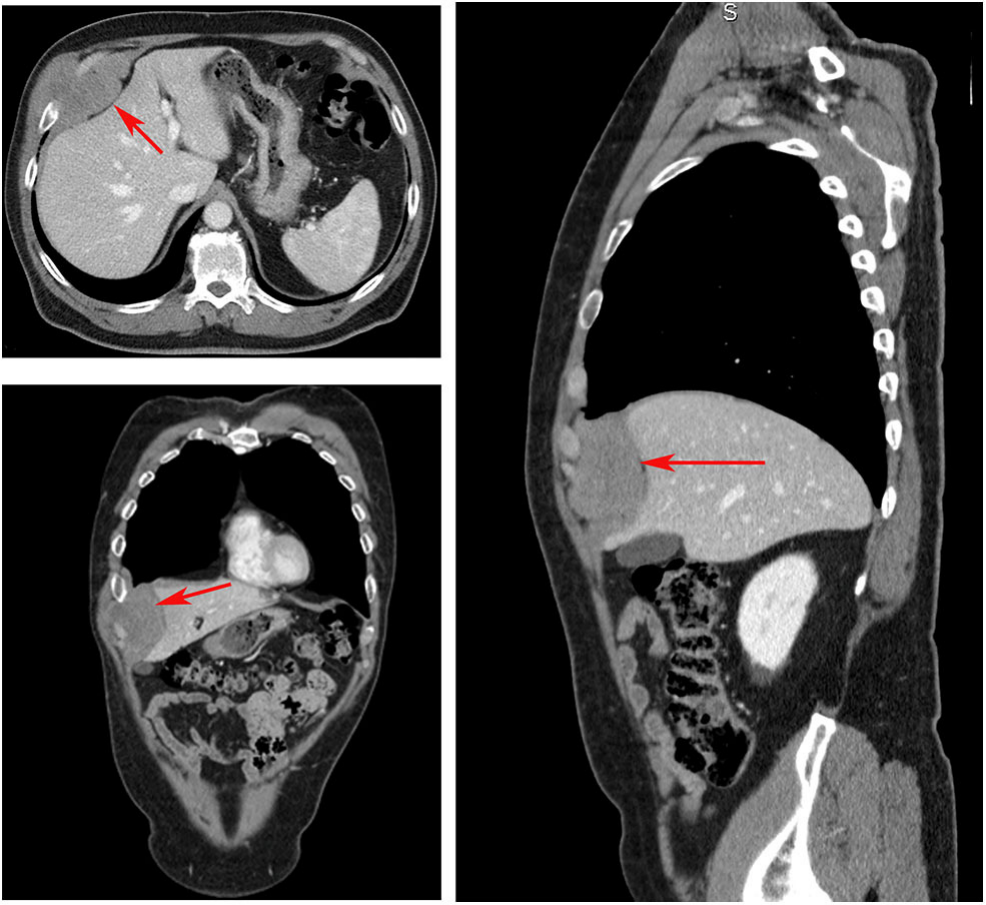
Computed tomography scan showing a homogenous, solid tissue, dense mass (red arrows) of 80 mm × 62 mm, in the anterobasal region of the right hemothorax involving the anterior arch of the seventh, eighth, and ninth ribs and their cartilages.
